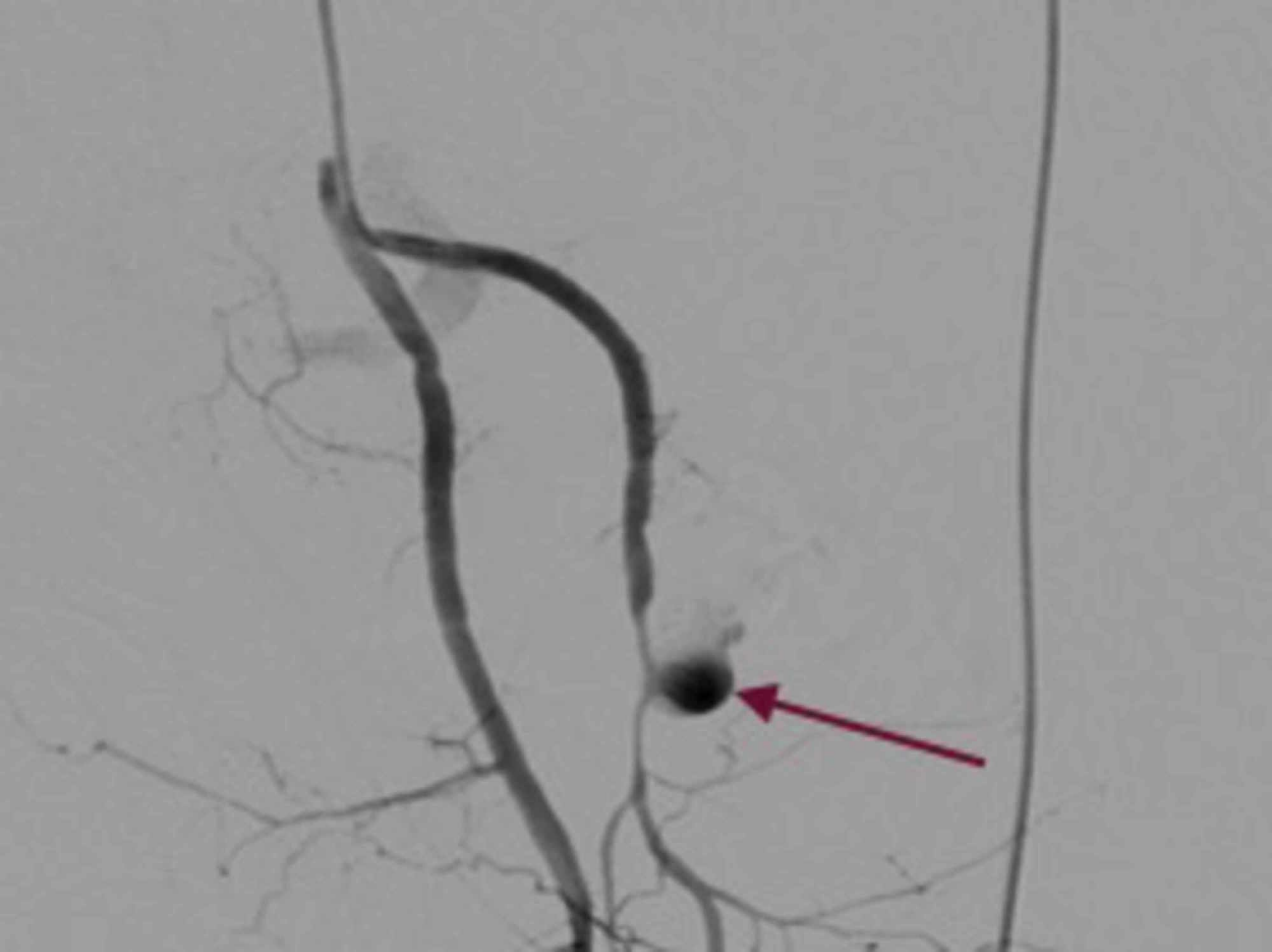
Computed tomography (CT) angiography showing right internal pudendal artery aneurysm (arrow)
